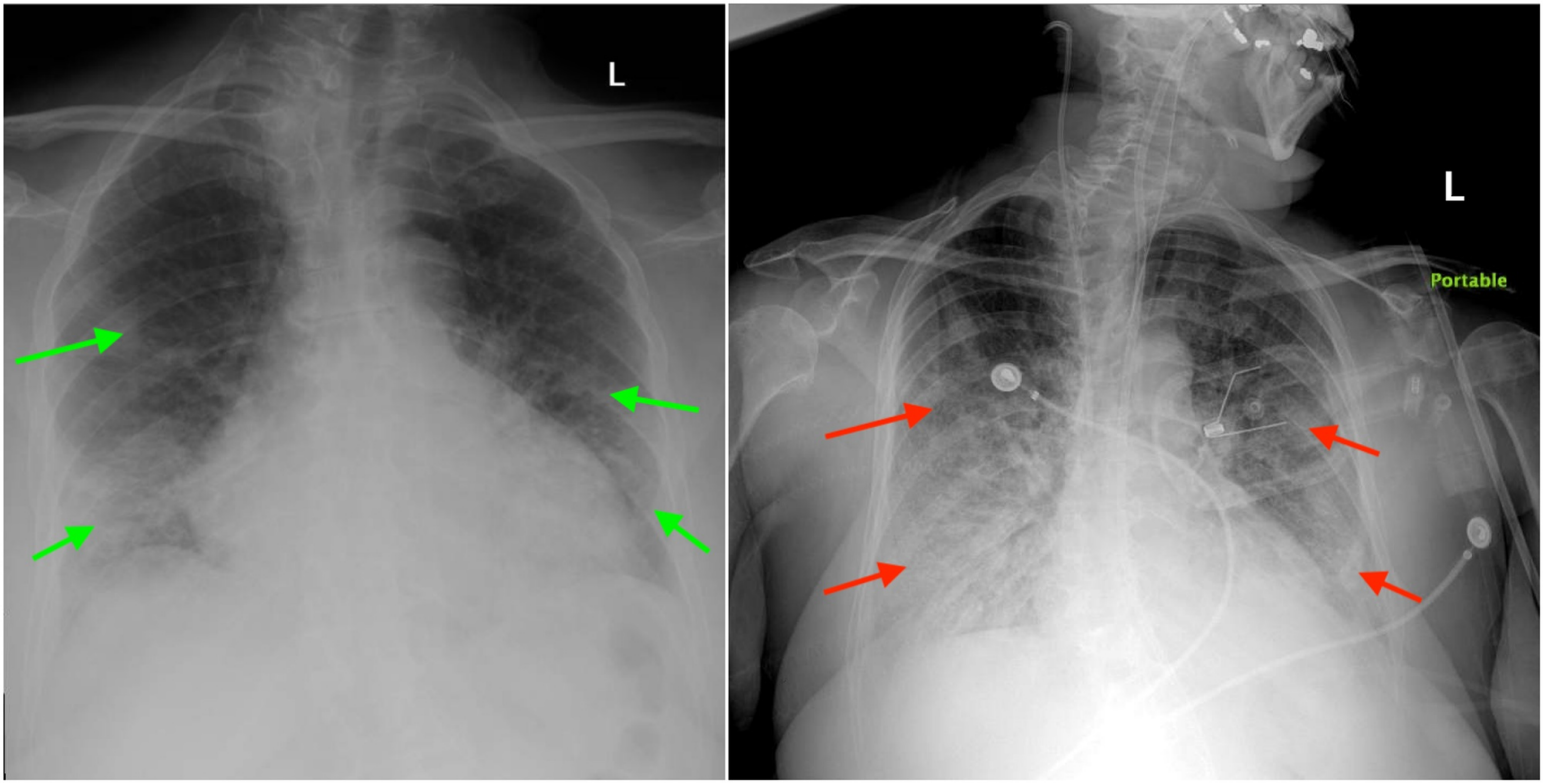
Initial chest x-ray showing bilateral airspace disease (left, green arrows). Post intubation chest x-ray on day three showing endotracheal tube and increased bilateral infiltrates in comparison to the chest x-ray on admission (right, red arrows)
